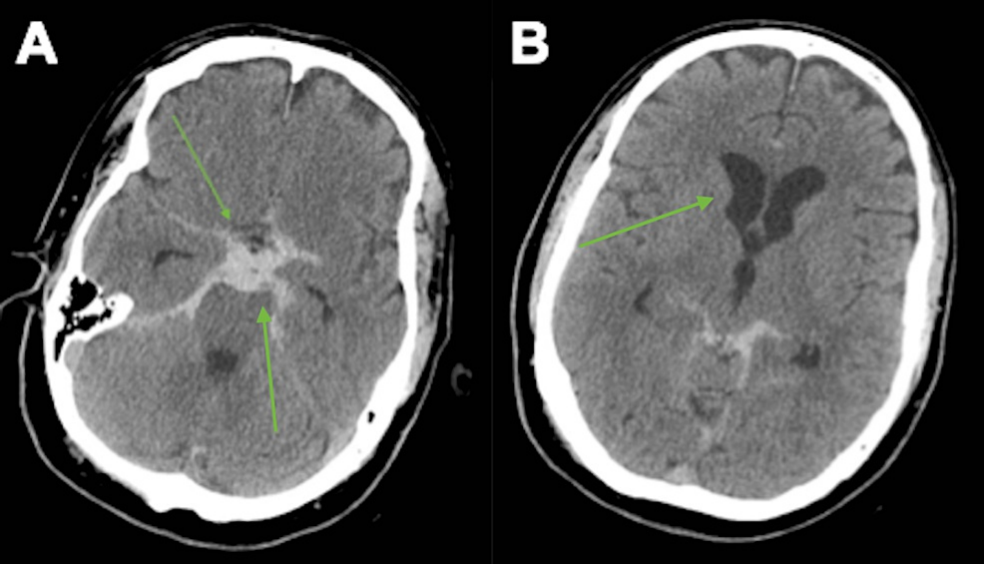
Axial computed tomography of the head demonstrating extensive diffuse subarachnoid hemorrhage involving the premedullary and prepontine cisterns extending into the basal cisterns (A) and evidence of early obstructive hydrocephalus (B)
